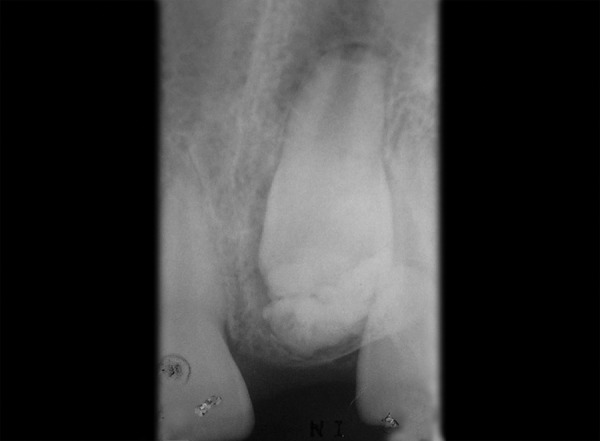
Intraoral periapical radiograph showing multilocular radiopacity enveloping the unerupted incisor with incomplete root formation
